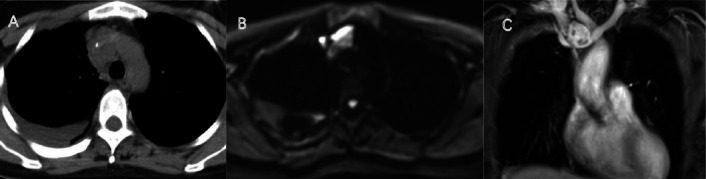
A 49-year-old woman showed a well-defined low-density mass in the right anterior superior mediastinum on axial CT with spotty calcifications (A); DWI sequence showed partial diffusion restriction (B), and enhanced MRI revealed heterogeneous enhancement with non-enhancing necrotic regions (C).
